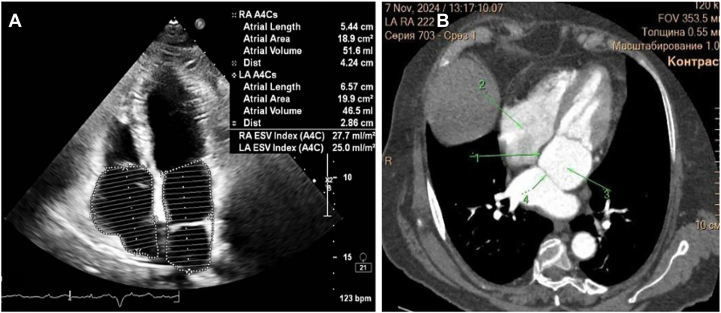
A: Transthoracic echocardiography 4-chamber position. B: Computer tomography of the heart with contrast. 1 = interatrial septum; 2 = right atrium; 3 = anterior-inferior compartment; 4 = fibromuscular membrane.
